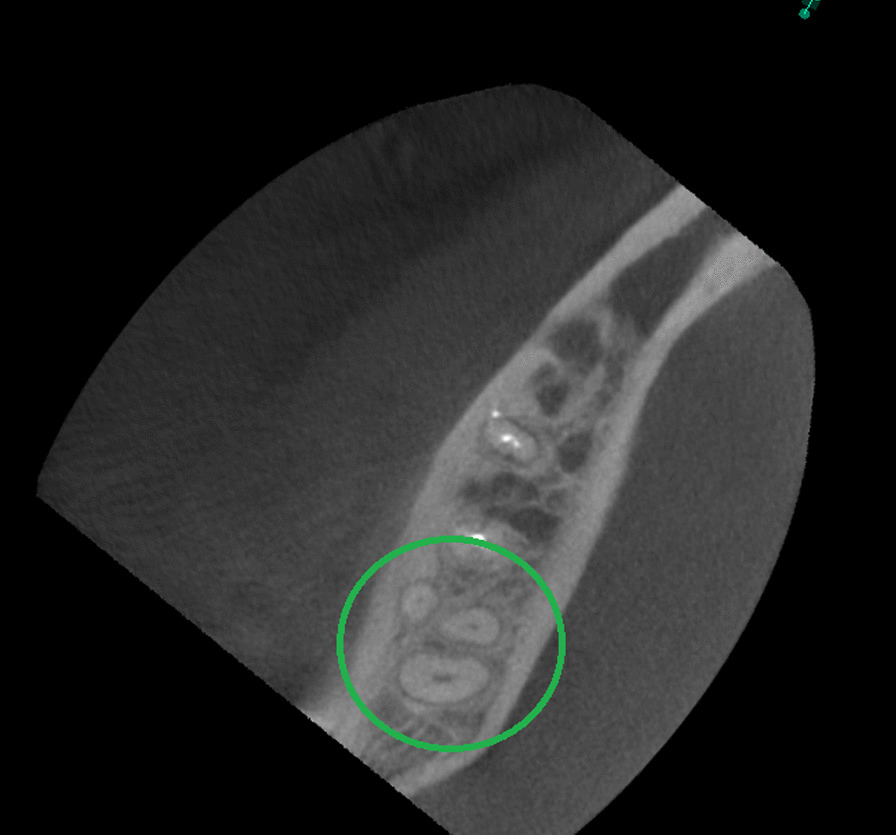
Axial view from CBCT scan showing mandibular second molar with three roots (Radix Paramolaris)
